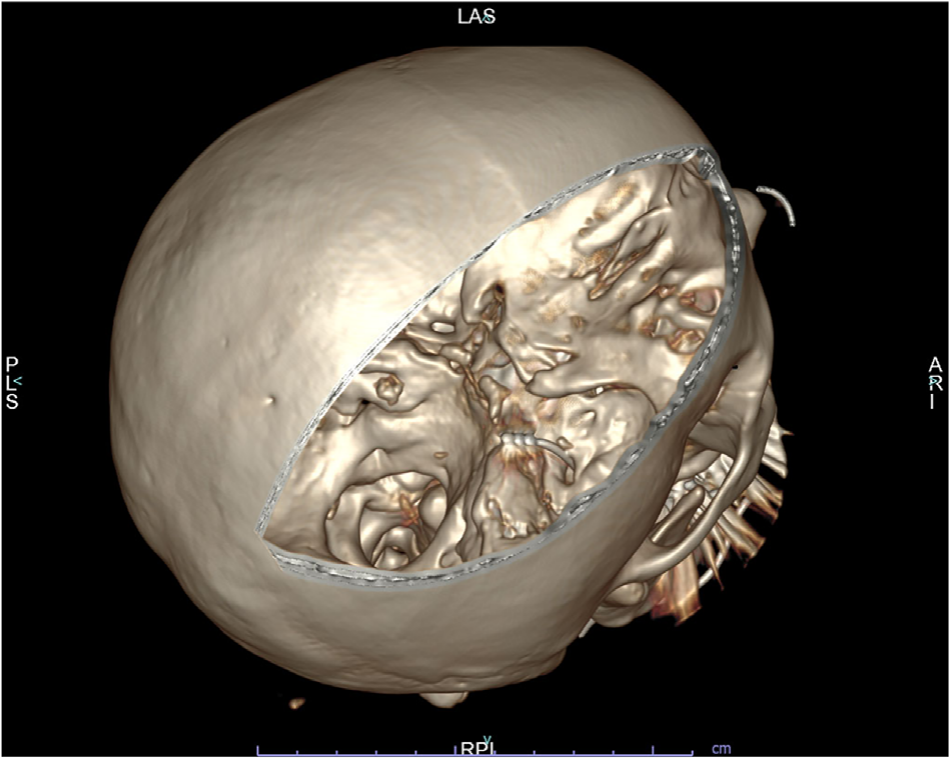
CT image reconstruction of the first case. Visualization of the tripolar electrode clearly bent over the gasserian ganglion
